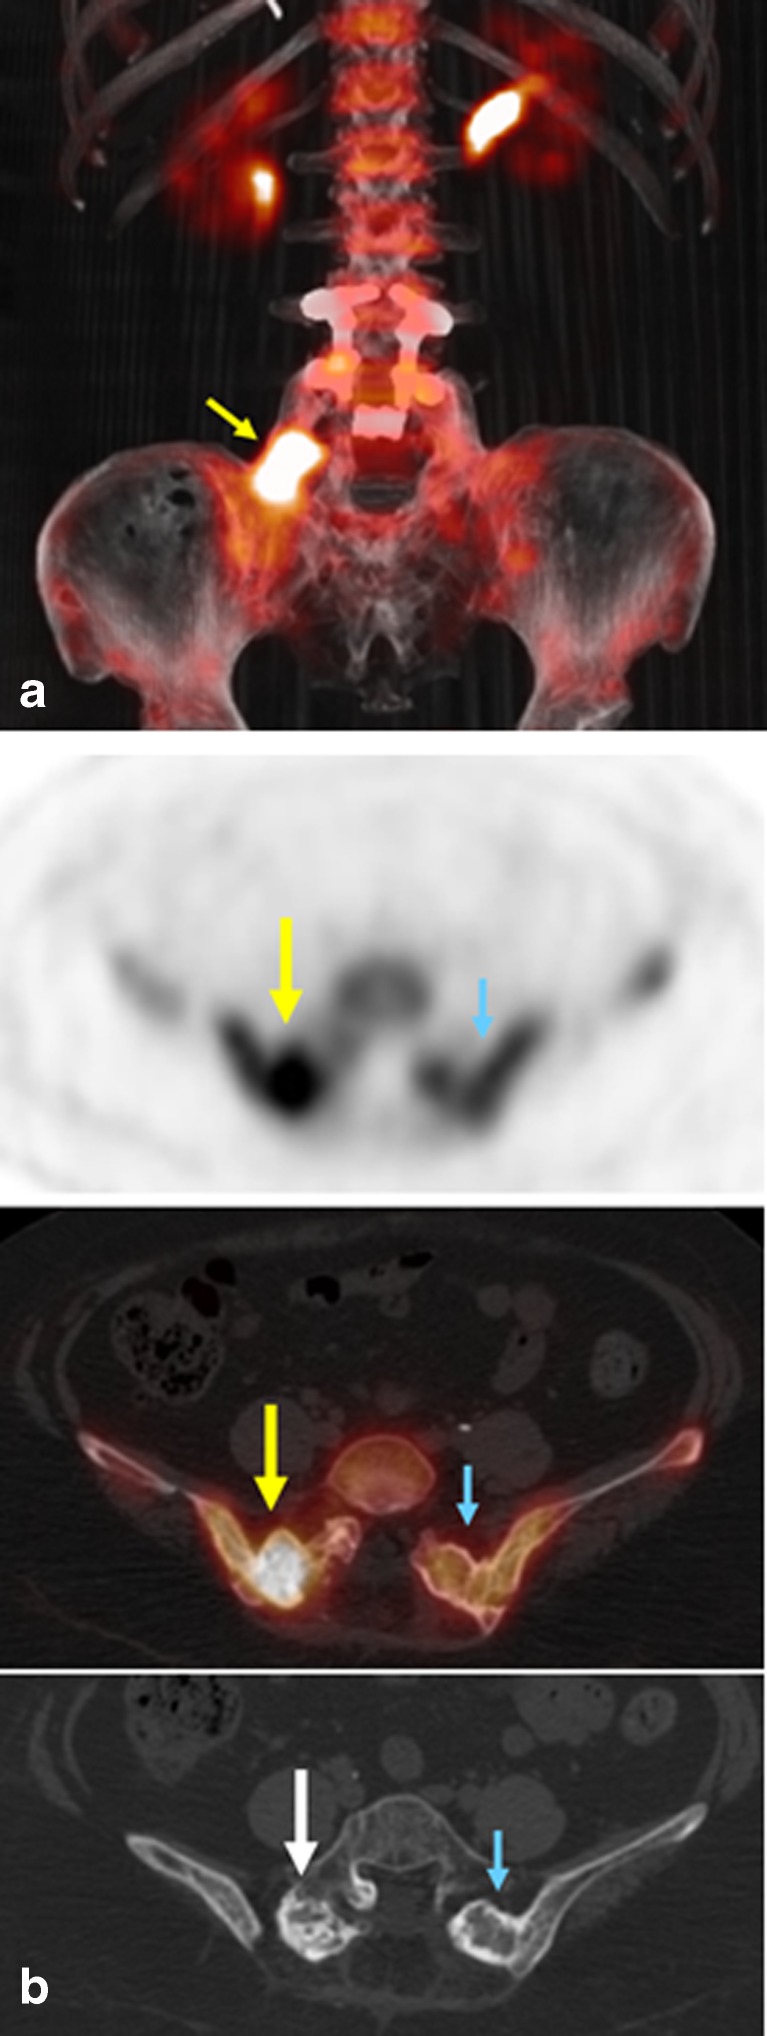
PET/CT images in patient 7. a Anterior view 3-D fusion PET/CT maximal intensity projection image demonstrates intensely abnormal activity at the right side of L5-S1 (*yellow arrow*) that was confirmed to be bone graft instability requiring surgical revision. b PET, PET/CT fusion, and CT images show markedly asymmetric activity (*yellow arrows*) at the right L5-S1 lamina and facet which is the site of a prior bone graft. Relatively normal activity is apparent within the bone graft located on the contralateral side (*blue arrows*). Increased sclerosis is apparent on the CT image (*white arrow*) at the right L5-S1 graft compared to the left that was initially described as postoperative changes (and difficult to differentiate from graft failure) on the initial CT scan
